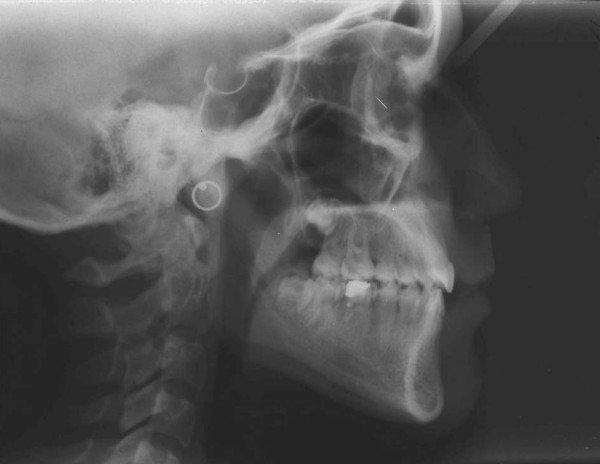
Posttreatment cephalometric radiograph of the patient.
